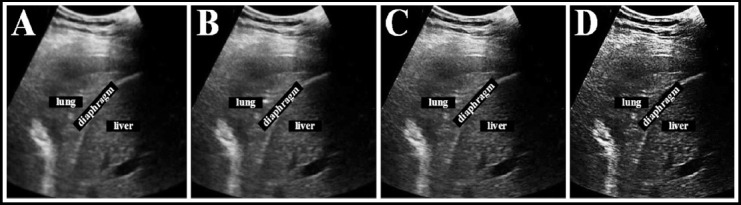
Comparison of the reconstruction effects of three algorithms on a male neonate’s lung ultrasound image (age 10 days, weight 3.17kg). *Note:* A was the original image; B was the WFFSF algorithm; C was the SNRP-FSF algorithm; D was the SDSS algorithm.
